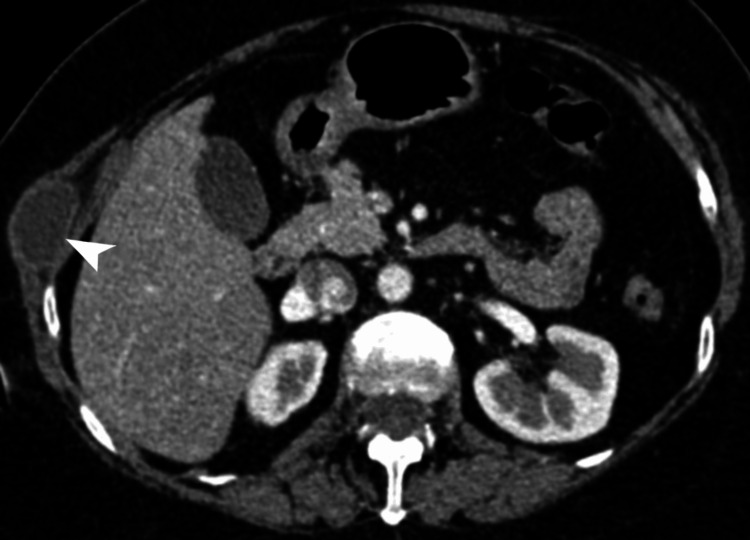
Right chest wall cystic lesion. Axial contrast-enhanced CT of the abdomen, in the soft tissue window, demonstrates a well-defined cystic lesion (white arrowhead) with peripheral enhancement, measuring 3.3 × 2.4 cm, in the right chest wall. CT: computed tomography
